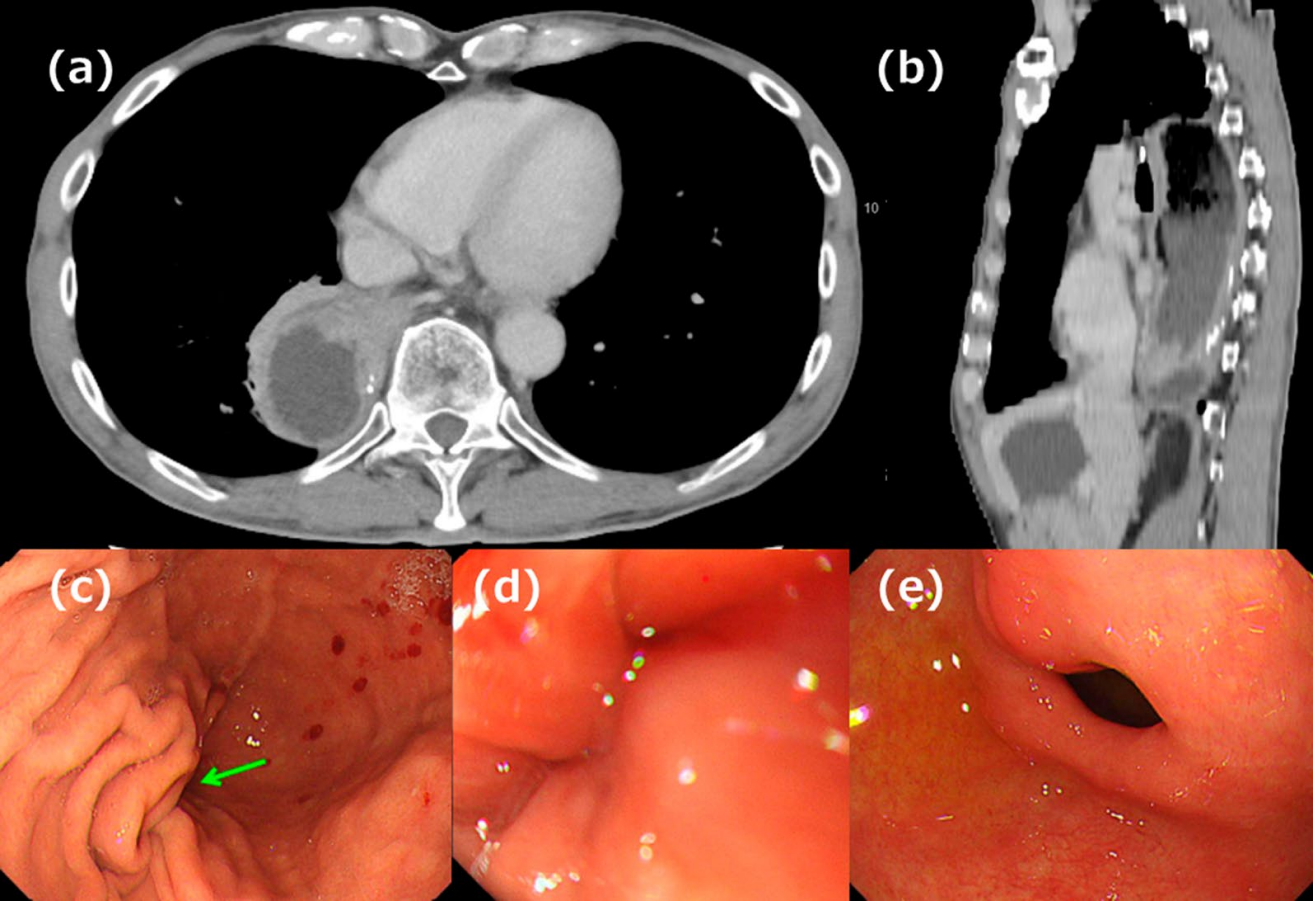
Computed tomography (CT) and esophagogastroduodenoscopy (EGD) findings. a, b Abdominal contrast-enhanced computed tomography reveals that the gastric conduit is flexed with dorsal and caudal deflection within the mediastinum. c A sharp angulation of the gastric conduit was noted in the distal portion, as indicated by the green arrow. d A twisting of the mucosa is observed. e No evidence of pyloric stenosis is identified
